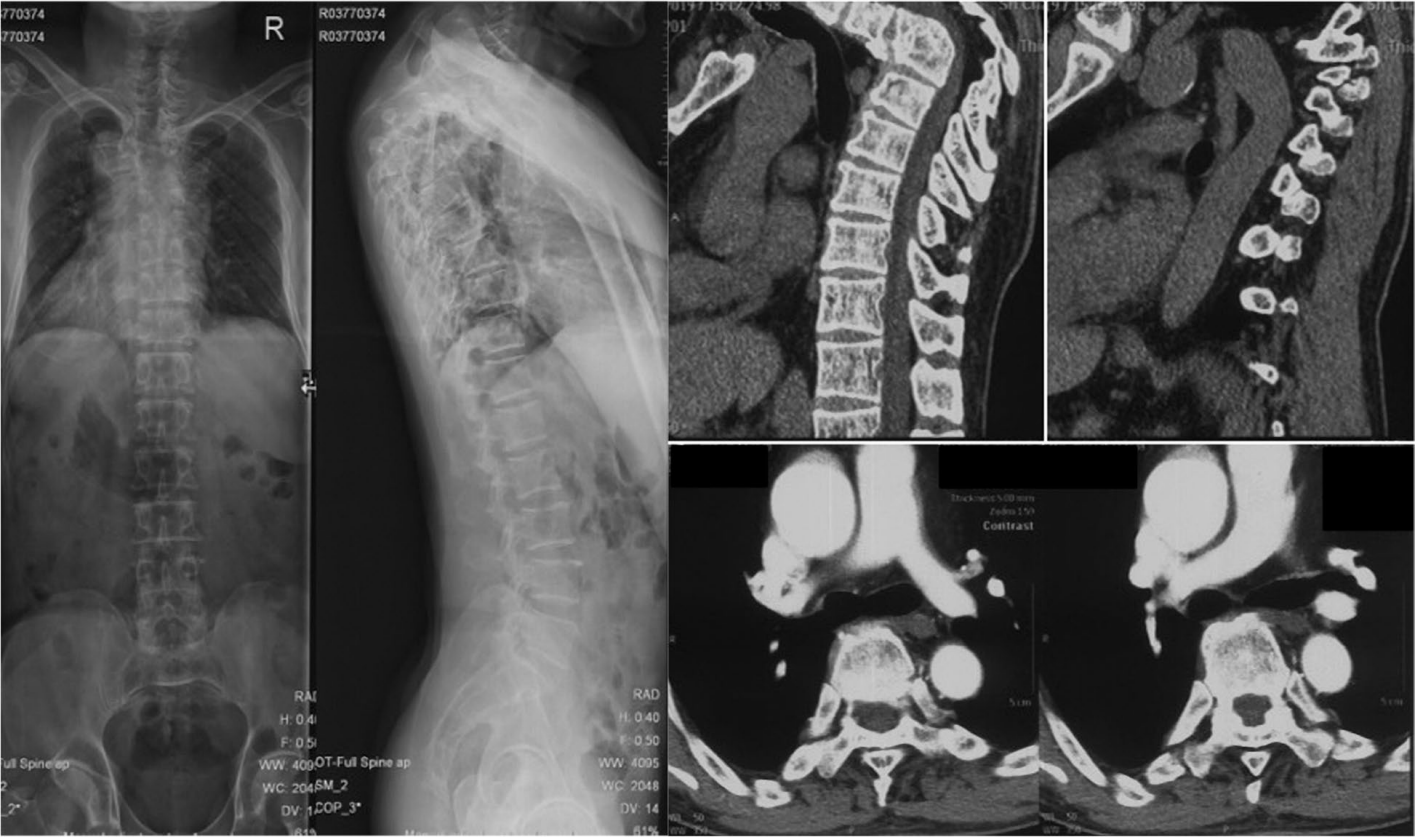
A 53 years old patient presented with back pain and shortness of breath. The kyphotic angle of T3-11 was 79.2° and SVA was measured 2.2 cm. CT scans showed that the aorta was seen near the lateral-wall of the apex vertebra and in front of the costal head (Grade 2)
